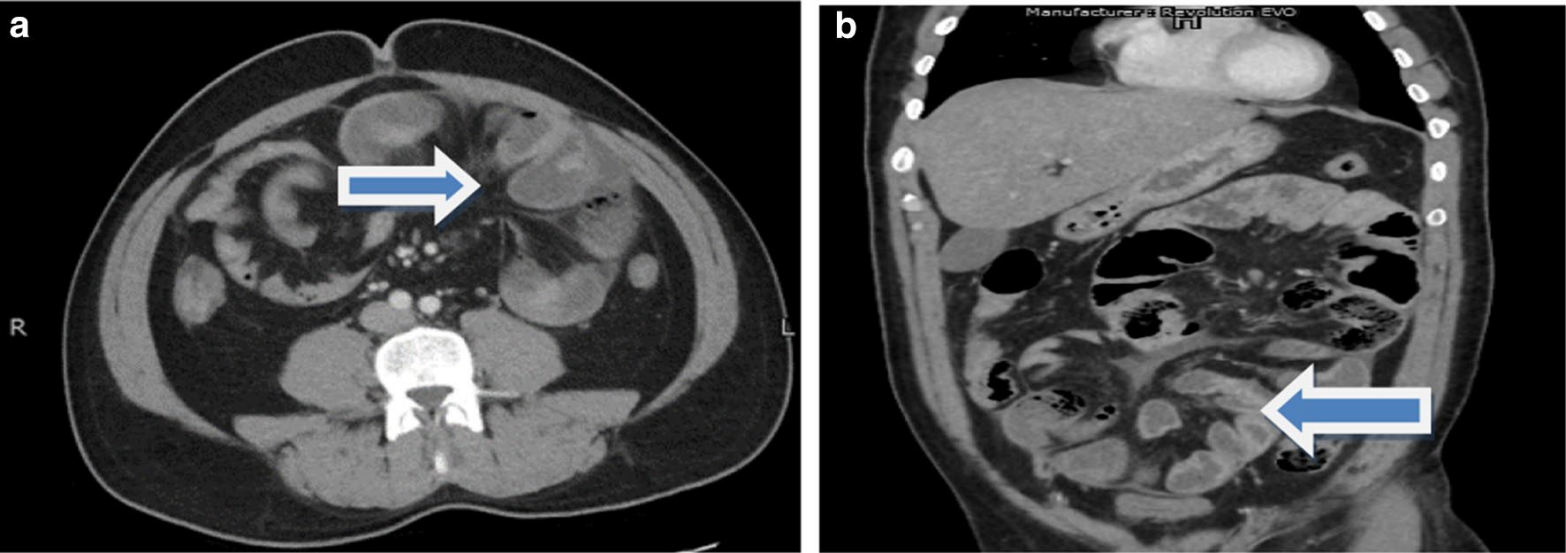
a and b Axial and coronal views of contrast-enhanced abdominal CT scan in which arrow shows twisted bowel loops raising the possibility of internal herniation
